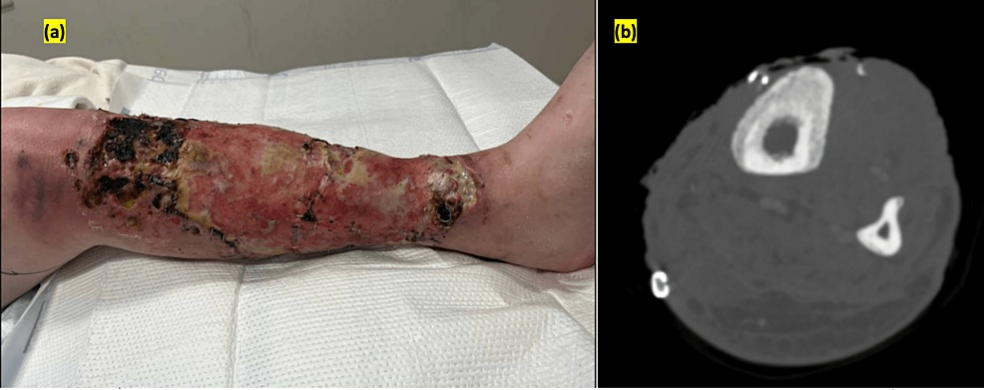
(a) Image of the left lower extremity cellulitis. (b) Contrast-enhanced computed tomography scan of the left lower extremity showing an underlying periosteal reaction, erosive changes, and cortical thickening along the left tibial shaft, suggestive of osteomyelitis.
